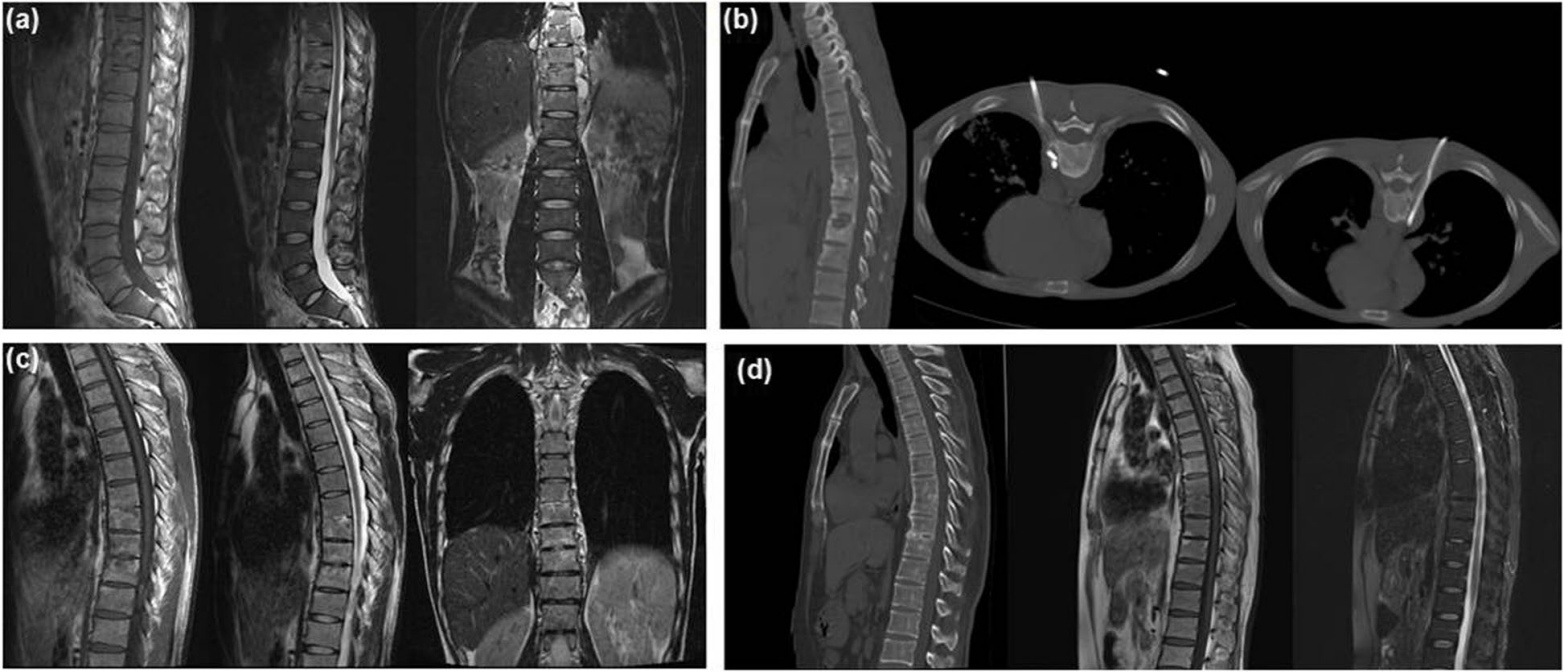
a Sagittal and coronal MRI images showing a 31-year-old male with mild spinal tuberculosis at the T8 ~ T11 levels. Tuberculosis accumulated in the vertebral body and intervertebral space with a smaller paravertebral abscess; no distinct kyphosis was observed. b Sagittal and axial CT scan showing tuberculosis of the thoracic vertebra. An abscess was treated with CT puncture, followed by two months of drainage.c At one year follow-up, sagittal and coronal MRI showed significant bone destruction and intervertebral space reduction, but the tuberculosis abscess has disappeared. d Four years after combined treatment with CT guided puncture, catheter drainage and 18 months of standard chemotherapy, sagittal CT and MRI showed a solid fusion at the T8/T9, T10/11 levels and destruction of the thoracic vertebrae. The paravertebral abscesses had disappeared at the T8 ~ T11 levels, with no significant progression of local kyphosis.
